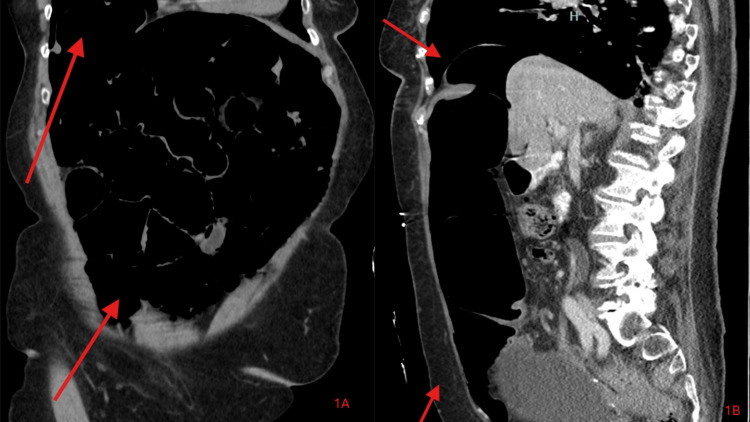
Computed tomography of abdomen and pelvis (A, B). Pneumomediastinum and pneumoperitoneum, concerning for possible colonic perforation, though no clear area of perforation was noted on imaging. Arrows represent air found in the mediastinum and peritoneum.
